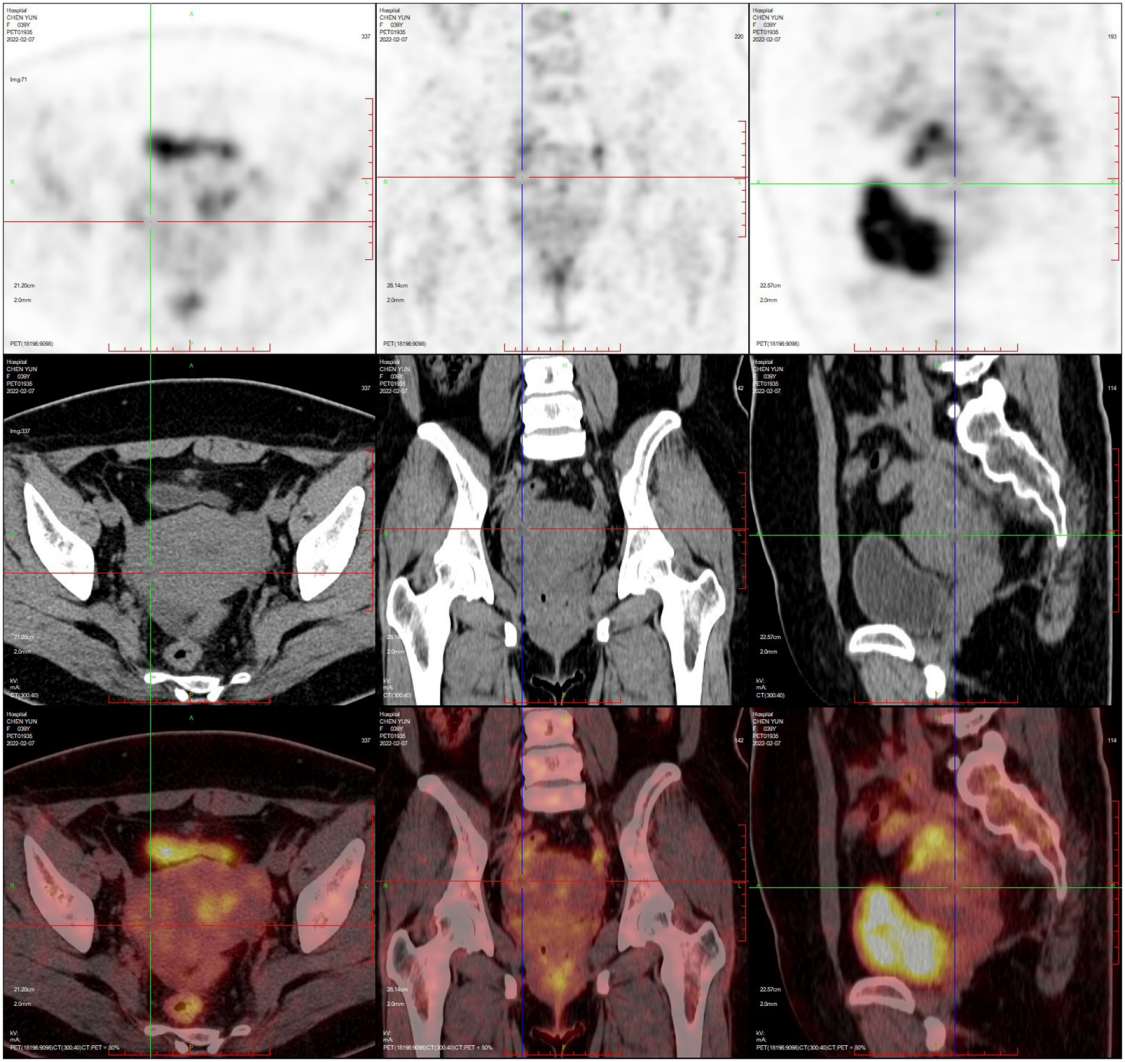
PET-CT after ovarian lymphoma surgery (Case 2).
